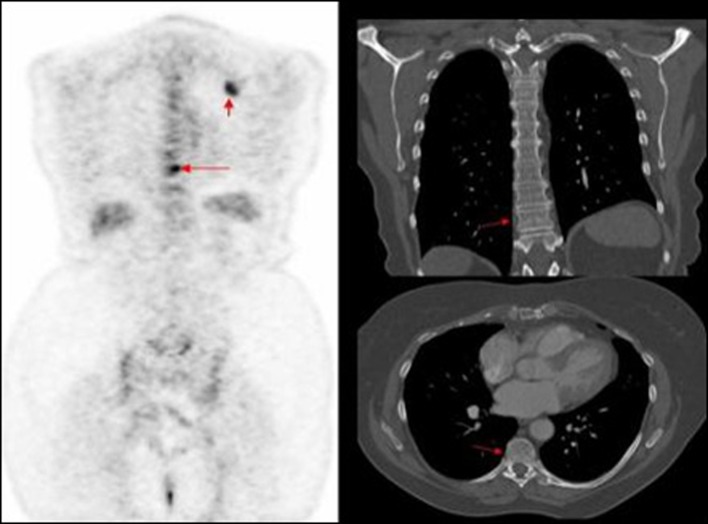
PET image of thoracic vertebrae and left scapula metastatic deposits, not identified on corresponding CT.
